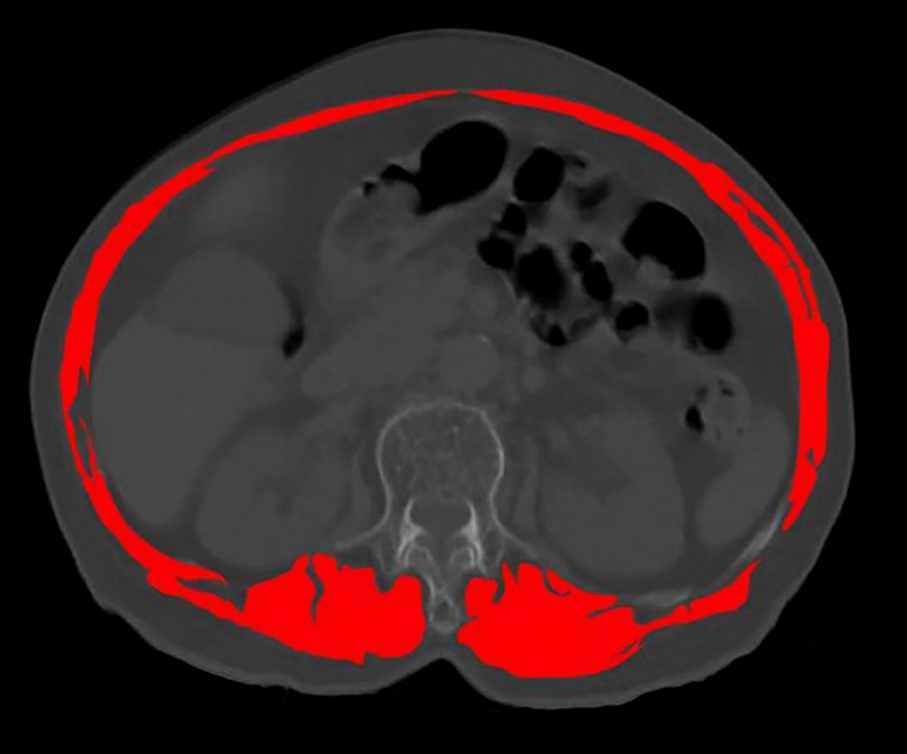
Calculate the muscle area On the CT image at the level of the pedicle of the thoracic 12 vertebrae
